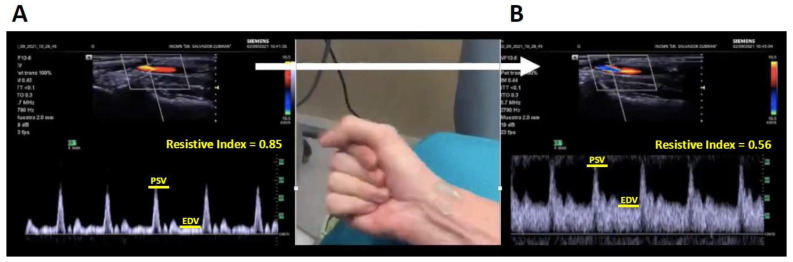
Pulsed-wave Doppler image of radial artery: (A) Doppler signal at baseline and (B) Doppler signal after a maneuver to recruit blood flow to the hand muscles. PSV = peak systolic velocity; and EDV = end-diastolic velocity. The resistive index is calculated as (PSV − EDV)/PSV.
